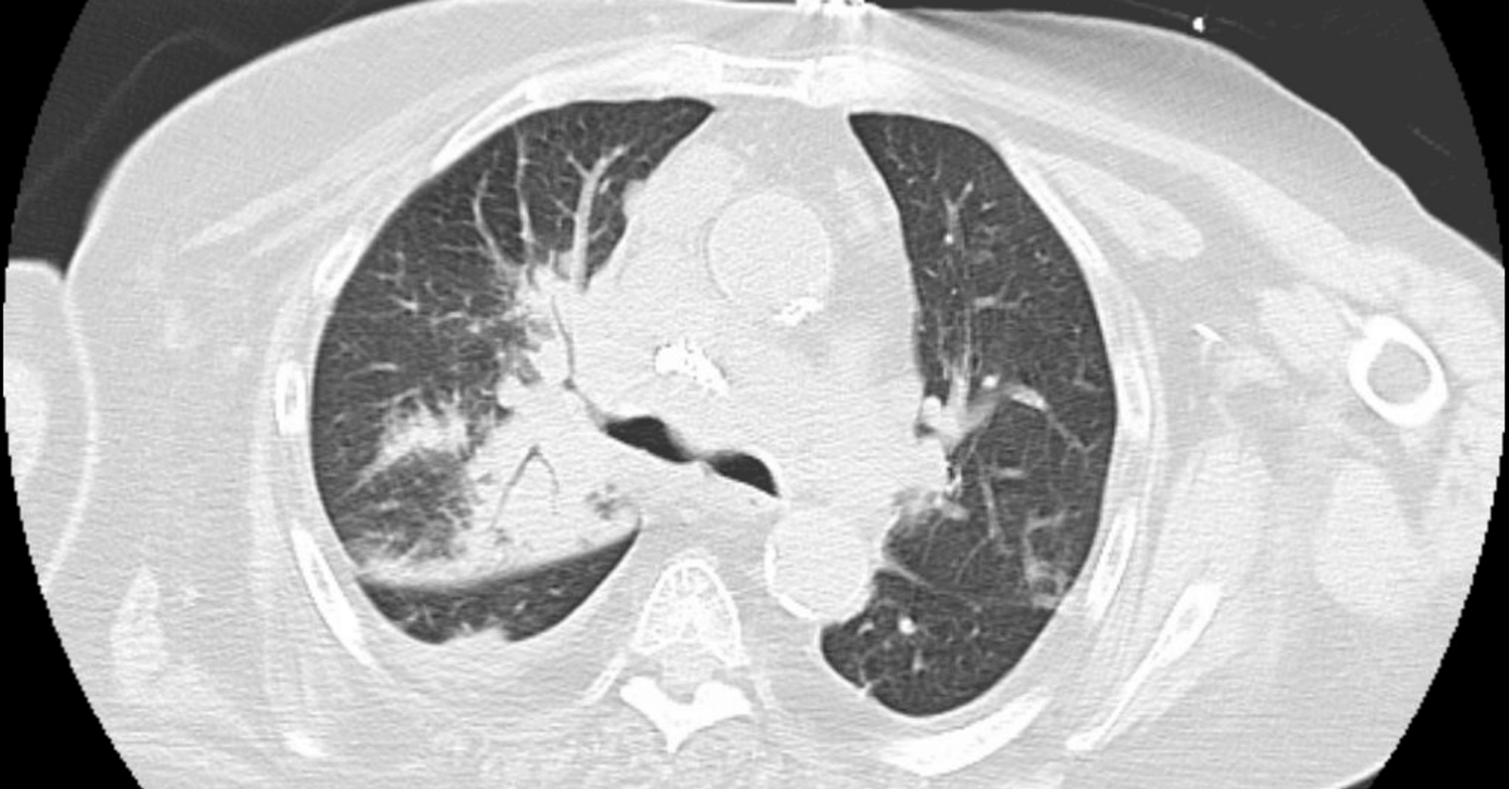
CT chest with a lung view Right hilar mass and adjacent consolidation concerning for a combination of pneumonia and interstitial edema due to lymphatic obstruction (worse than prior). Findings are consistent with malignancy and metastatic disease.
